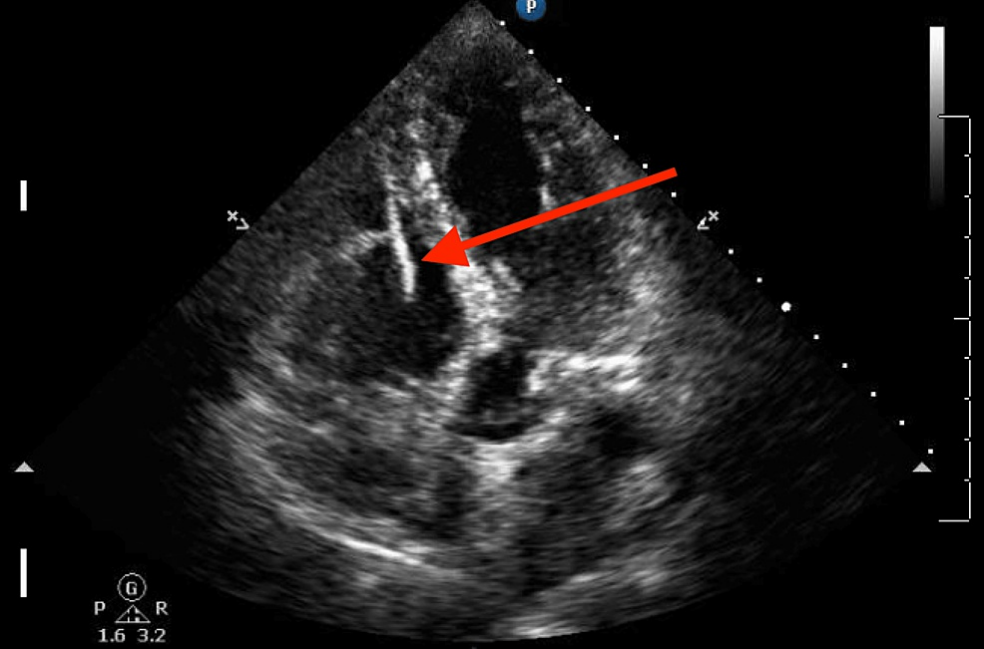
Transthoracic echocardiogram of cement embolus Transthoracic echocardiogram shows a linear, non-flexing, and immobile structure extending from the tricuspid annulus to nearly the right ventricular apex (red arrow). No adherent mass is seen.
